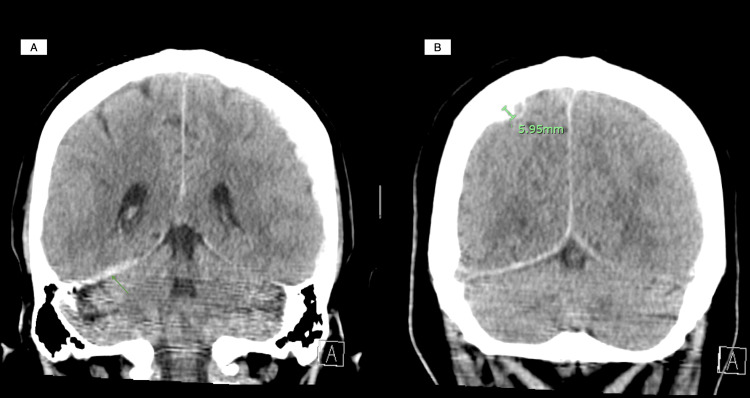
A non-contrast computed tomography (CT) of the brain (A) Acute trace subdural blood along the posterior falx and right tentorium; (B) Acute right convexity subdural hematoma measuring 5.95 mm.
